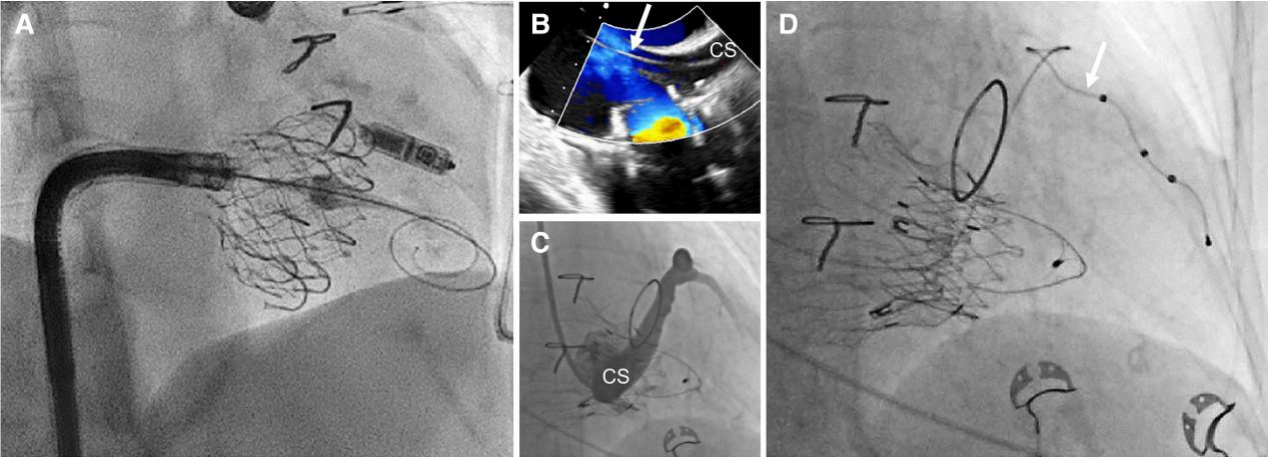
Examples of valve-sparing pacing strategies in the context of transcatheter tricuspid valve replacement. (*A*) Pre-emptive implantation of a LCPM before transcatheter tricuspid valve replacement with the EVOQUE system in a patient with a complete atrioventricular block during right heart catheterization. The position of the LCPM needs to be carefully chosen; (*B*) transoesophageal echocardiography guiding of coronary sinus lead implantation (white arrow) after transcatheter tricuspid valve replacement with the LUX valve; (*C*) sinus angiography highlighting potential interaction between the bioprosthesis and the coronary sinus; and (*D*) final pacemaker lead (white arrow) placement into the coronary sinus. CS, coronary sinus.
